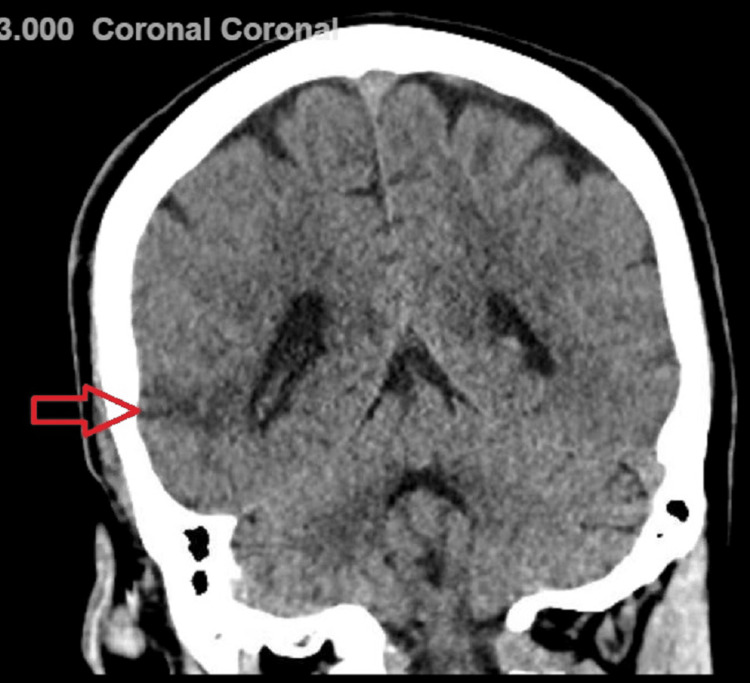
CT scan without contrast, coronal section, revealed indeterminate age infarct in the right temporal lobe (red arrow)
